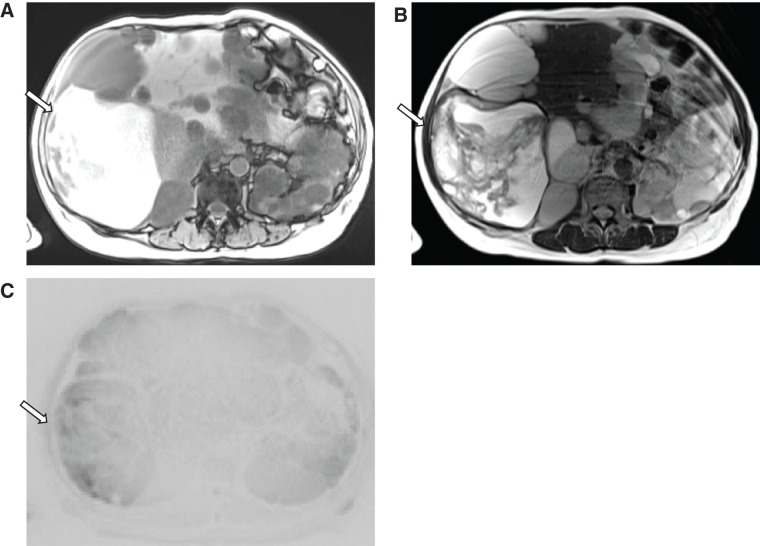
Preoperative MRI images. Abdominal MRI revealed multiple hepatic cysts, the largest measuring 15 cm in diameter (arrow). The largest cyst showed heterogeneous high signal intensity on T1-weighted imaging (A), T2-weighted imaging (B), and diffusion-weighted imaging (C).
